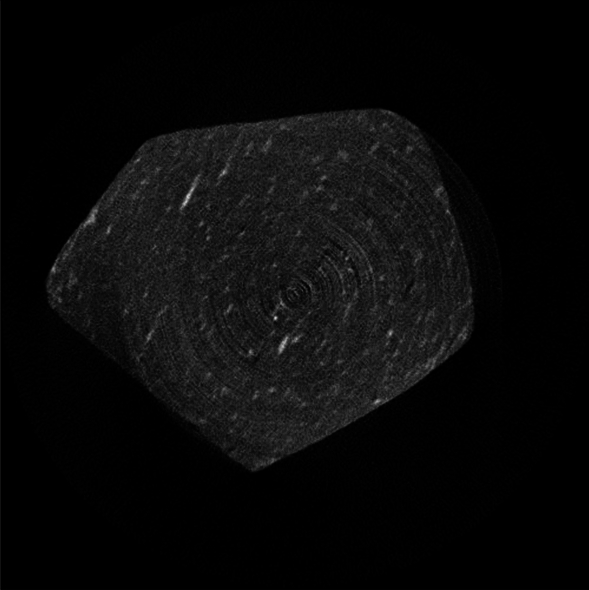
CT single-slice cross-sectional image of the sample in scheme 1.
